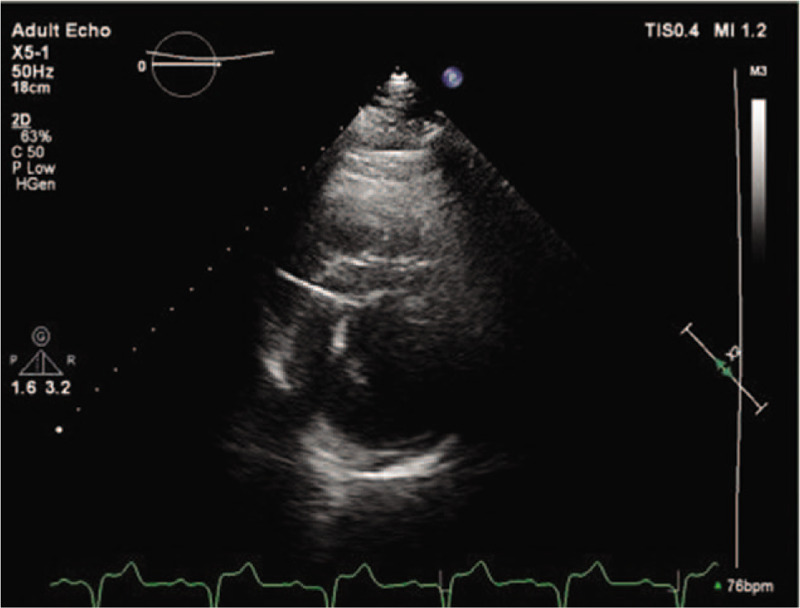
Echocardiographic parasternal short axis view shows the active fixation lead penetrating up to 10 mm into the interventricular septum, almost reaching the left ventricular endocardium.
